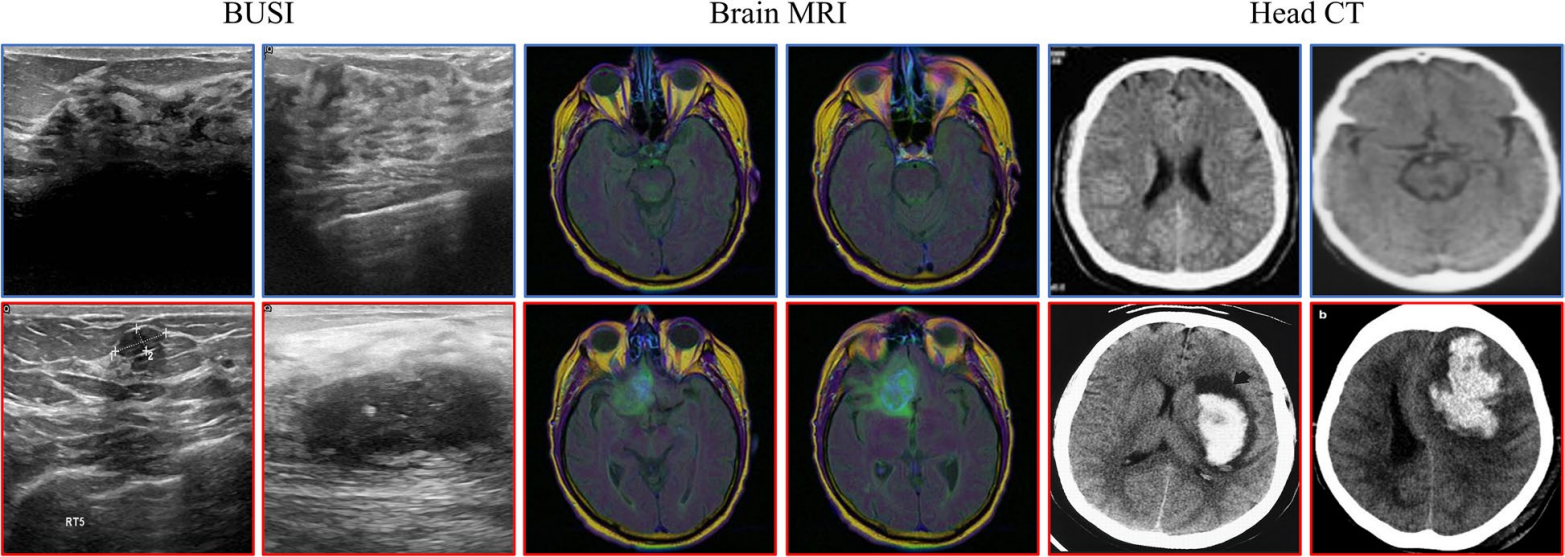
Example of a medical anomaly detection dataset. The first row shows examples of normal samples (framed in blue), while the second row shows examples of abnormal samples (framed in red). The first two columns are from the breast ultrasound images dataset (BUSI), the following four columns are from the brain magnetic resonance imaging (MRI) dataset, and the last six columns are from the head computed tomography (CT) dataset
